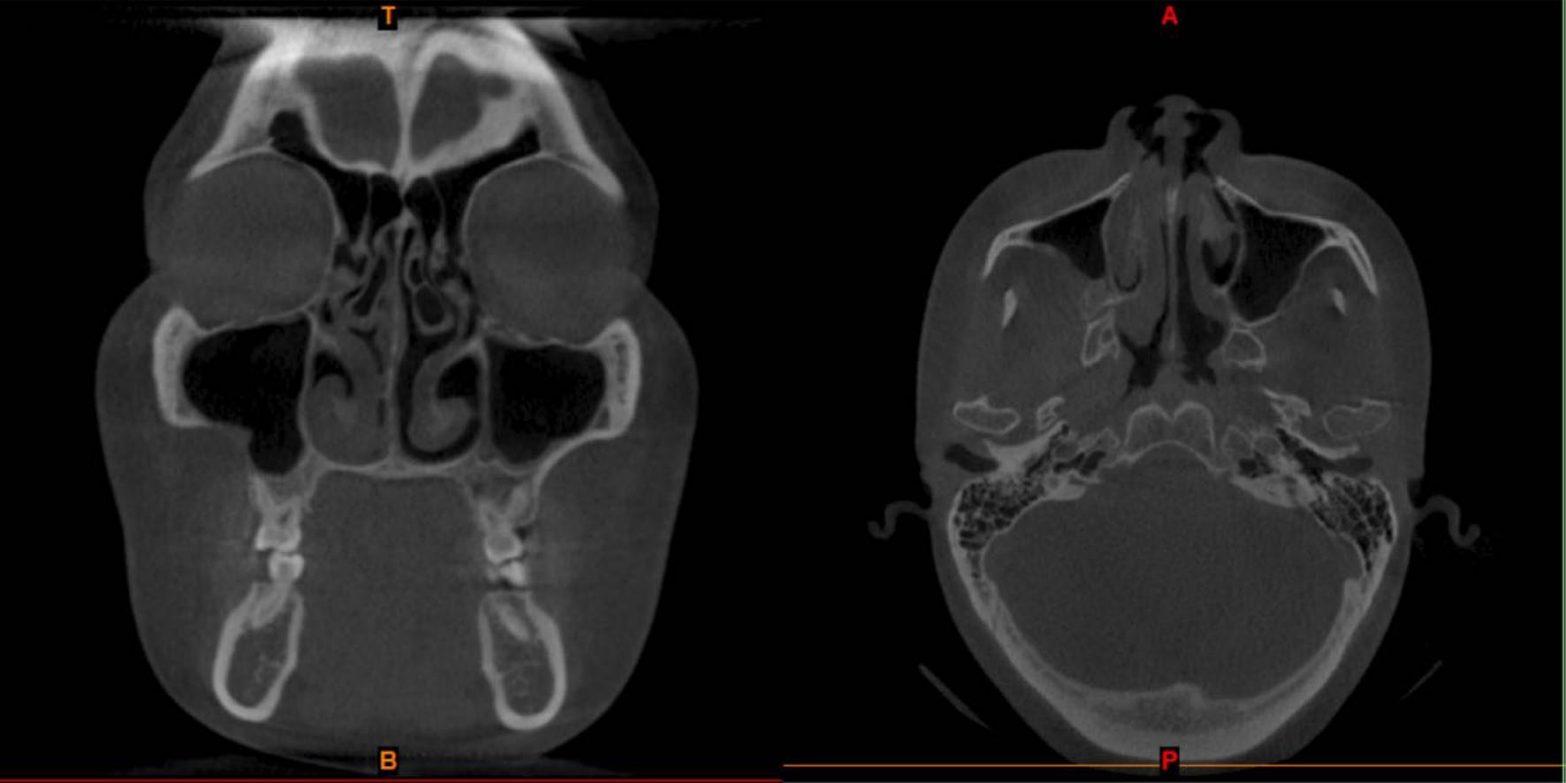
Post-operative CT scan showing a clear maxillary sinus at 12 weeks in the same patient (Figs. 1, 2, 3 and this figure is the same patient).
